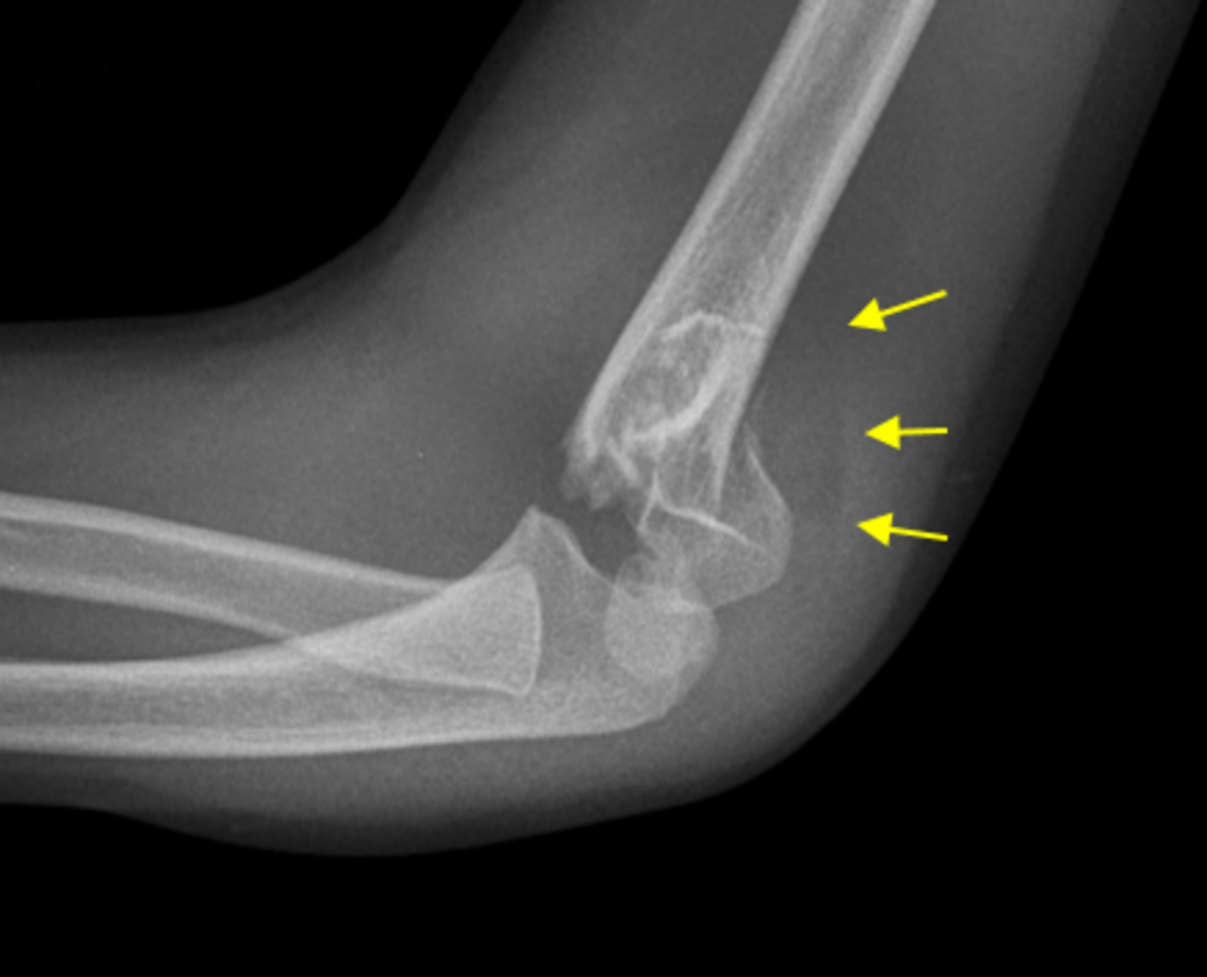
Lateral X-ray elbow projection of the patient in Figure 1 Arrows indicate a posterior fat pad sign.
